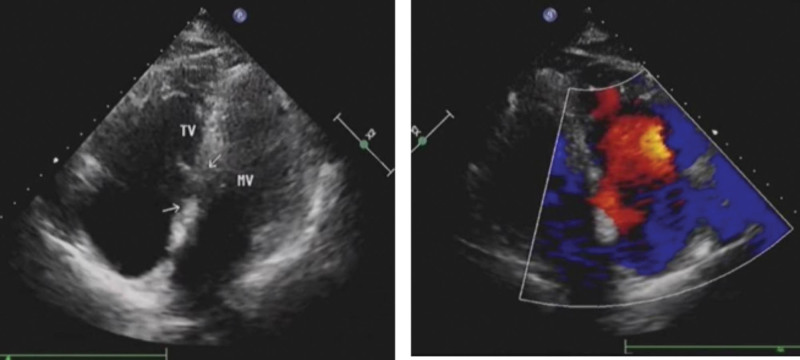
Admission echocardiogram of the patient. (A) Displacement of the tricuspid valve accompanied by atrial defect. (B) The position of Micra AV. (C) The position of AAI lead.
